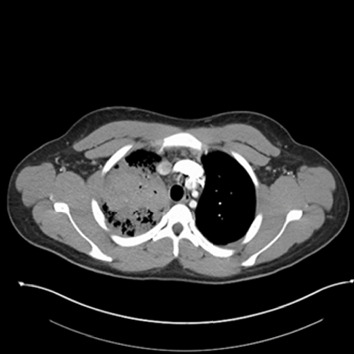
CT chest imaging. Patchy ground-glass consolidations were also seen in the right lower lobe. Mild centrilobular ground-glass opacities were present in the right middle lobe and lingula.
